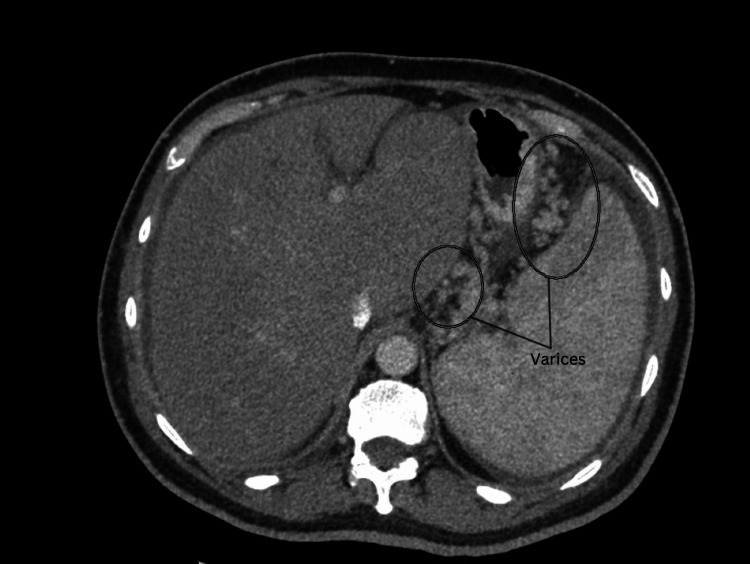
Abdominal CT image clearly showed the presence of varices (as indicated by the circles)
